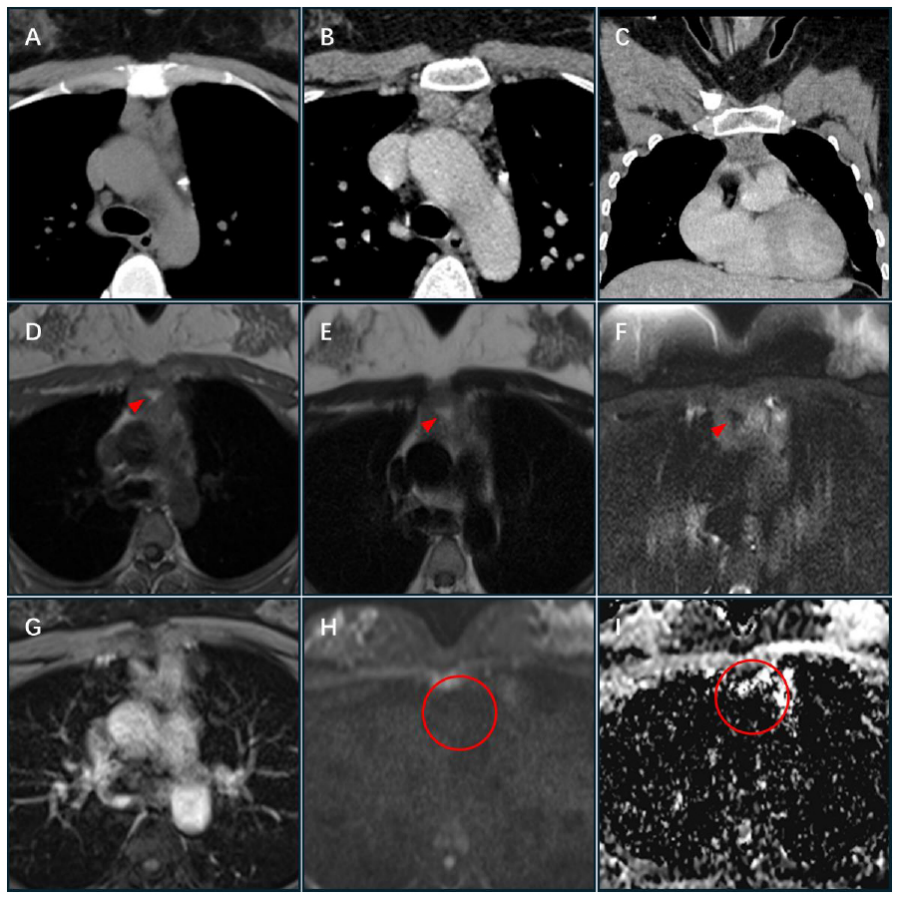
(A-C) Enhanced chest CT showed a nodular mass with mild enhancement located in the anterior superior mediastinum. (D, E) T1- and T2-weighted MRI showed a mass with low signal in the edge and a high signal in the center (arrow). (F) After fat-suppression sequence imaging, the central high signal area of the mass became low signal (arrow). (G) The enhanced scan showed significant enhancement. (H, I) The DWI sequence was a low signal, and the ADC sequence was a partially high signal (circle). CT: Computed tomography; MRI: Magnetic resonance imaging; DWI: Diffusion weighted imaging; ADC: Apparent diffusion coefficient.
